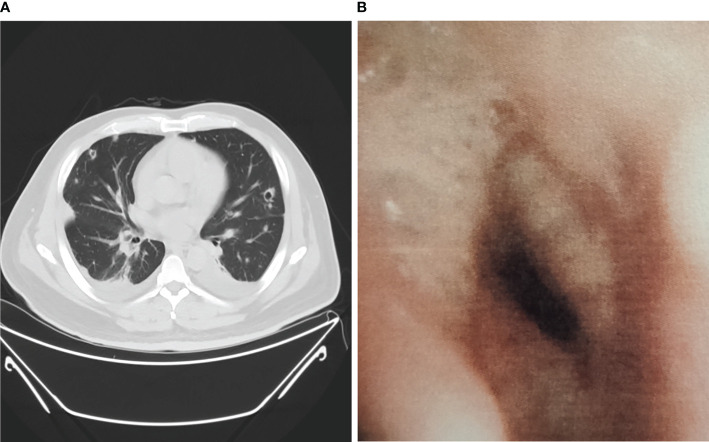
Chest CT scan and bronchoscopy of pulmonary aspergillosis in non-neutropenic patient. (A) Chest CT showed cavitation signs; (B) Bronchoscopy showed purulent secretions, mucosal hyperemia, and edema.
